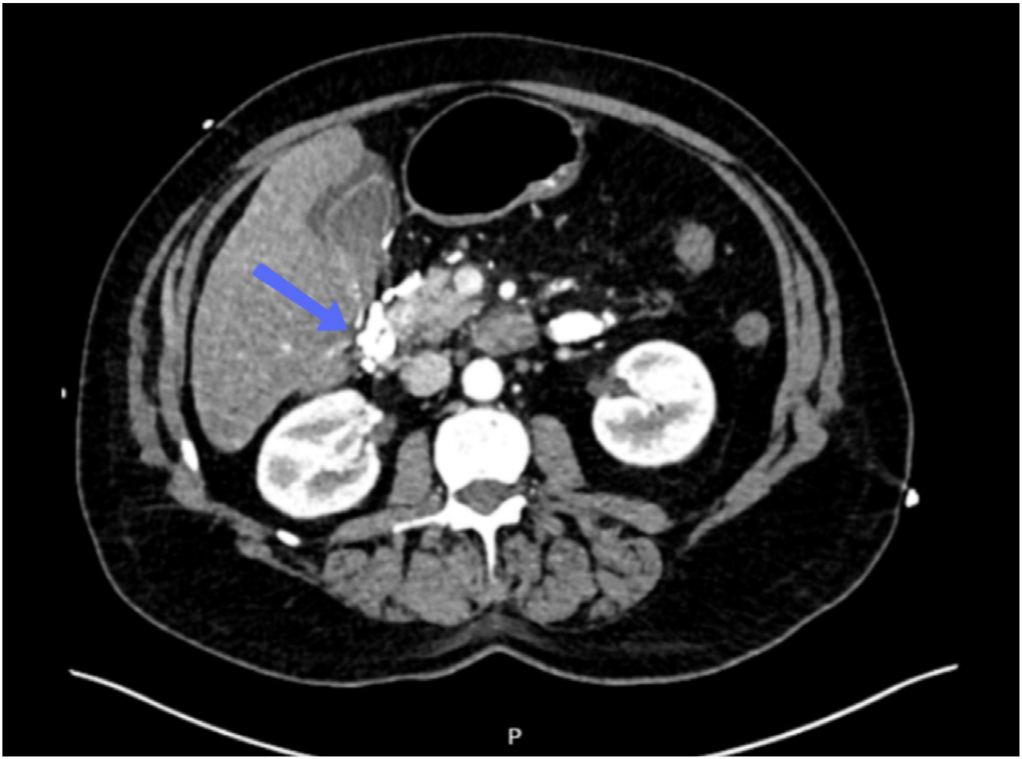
Postendotherapy computed tomographic scan showing Histoacryl-Lipiodol mixture at the site of the duodenal varix.
